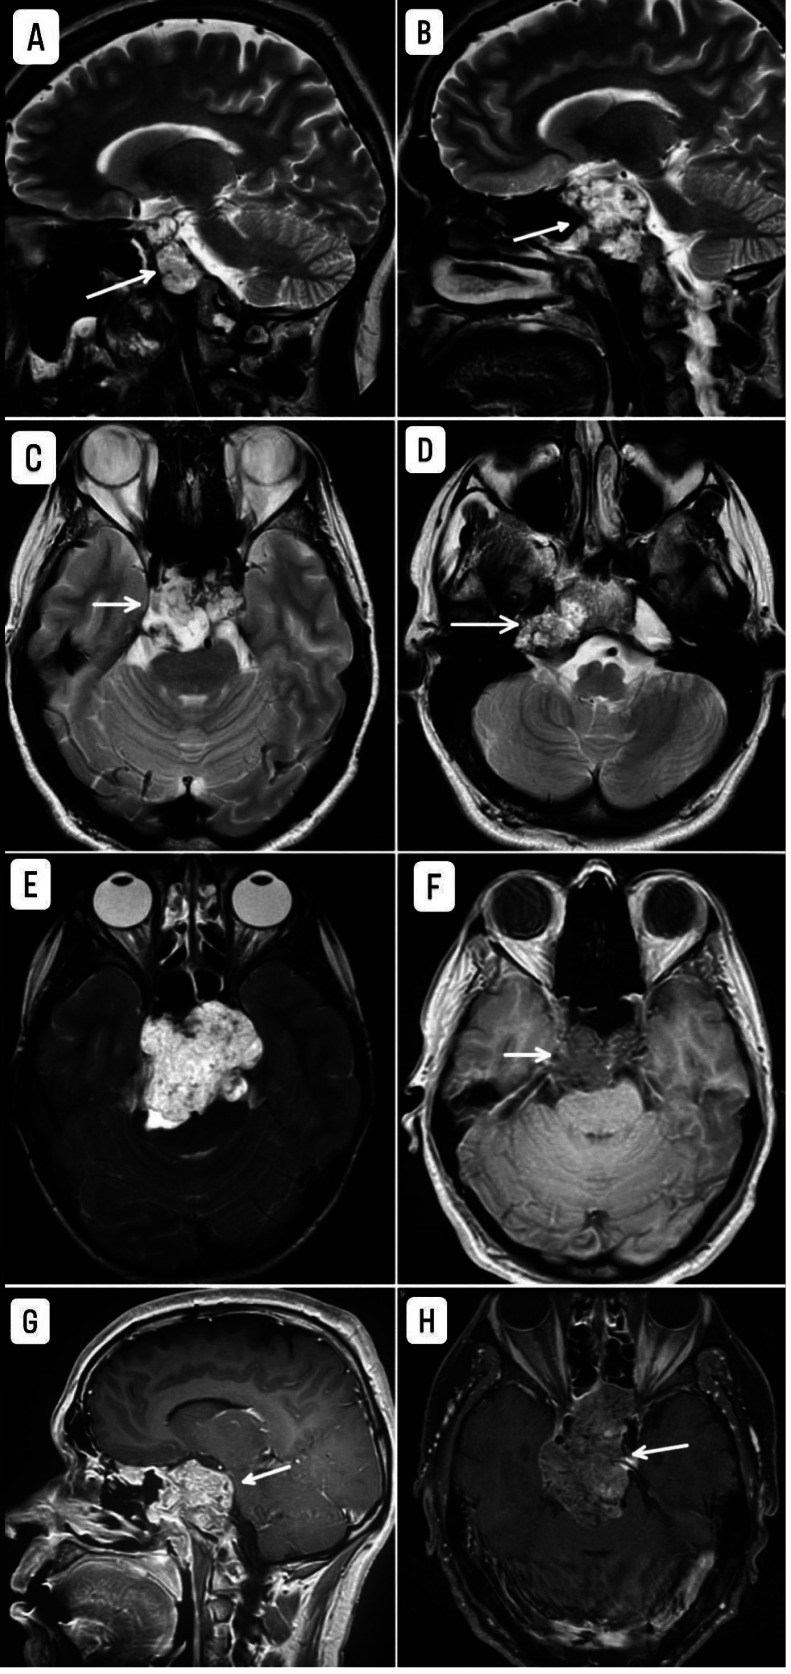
MRI findings of the skull base mass. (A, B) Sagittal T2-weighted images; (C, D, E) Axial T2-weighted images; (F) Axial T1-weighted image; (G) Sagittal T1-weighted image with contrast; (H) Sagittal T1-weighted image with contrast and fat saturation. The images depict an expansile mass lesion centered at the clivus and petrous apices, extending into the prepontine cistern, where it causes mild abutment of the right anterolateral pons and basilar artery. The lesion exhibits a heterogeneous T2 signal intensity and demonstrates heterogeneous contrast enhancement. There is bilateral cavernous sinus involvement, more pronounced on the left, where the lesion results in obliteration of the left Meckel’s cave. Superiorly, the mass extends to closely approximate the optic chiasm, raising concerns for potential visual pathway compromise.
